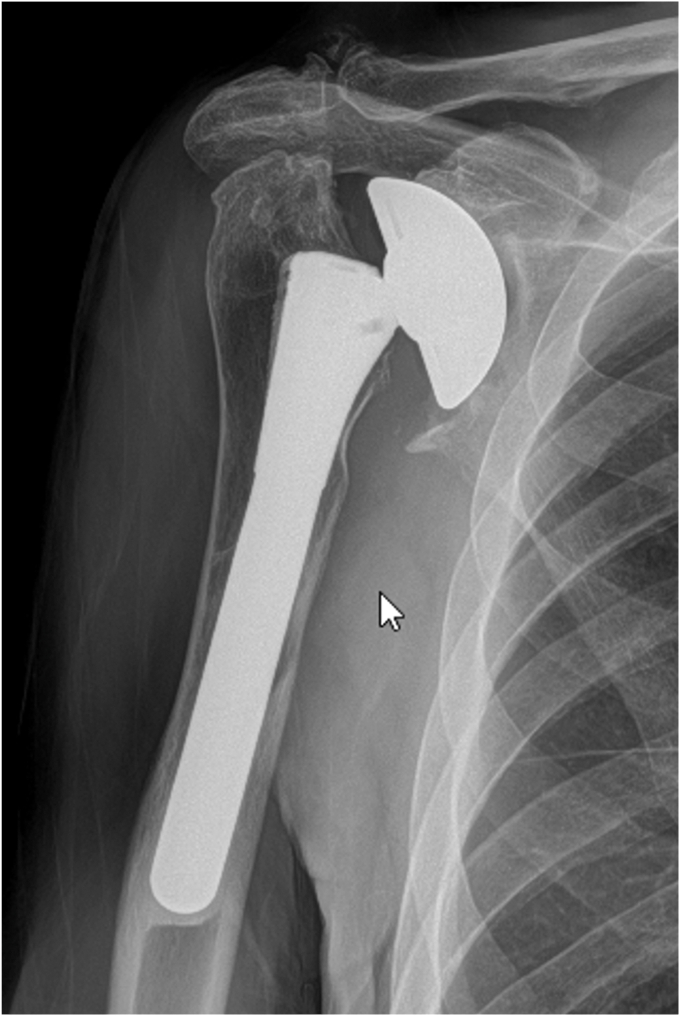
X-ray Grashey view demonstrating the preoperative right shoulder bipolar hemiarthroplasty with prominent glenoid bone loss.
